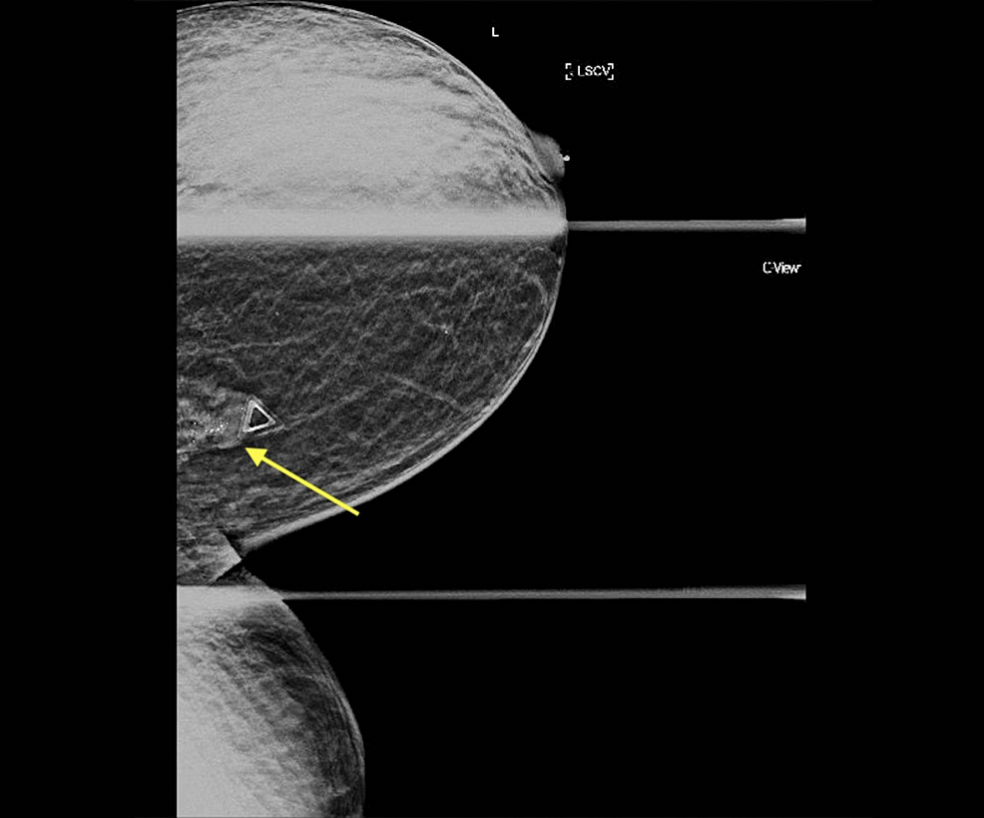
Diagnostic mammogram Ultrasound findings stated that "There is a mass with calcifications in the left breast upper inner aspect posterior depth. This correlates as palpated. No other significant masses, calcifications, or other findings are seen in either breast." Yellow arrow: mass with calcifications
